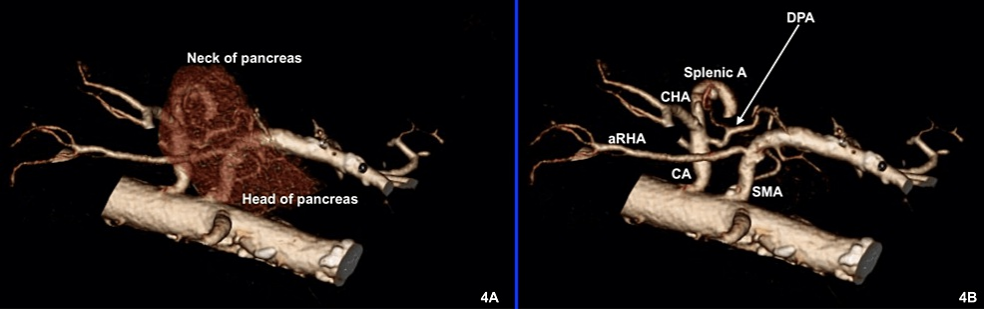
3D reconstruction of a CT scan image showing the course of both the DPA and the aRHA at the base of the mesopancreatic triangle. (A) Showing the relation of the DPA to the head of pancreas. (B) Demonstrating the relation of the DPA to the aRHA. SMA: Superior mesenteric artery; CA: celiac axis; aRHA: aberrant right hepatic artery; DPA: dorsal pancreatic artery; CHA: common hepatic artery; Splenic A: splenic artery; 3D: three-dimensional; CT: computed tomography
